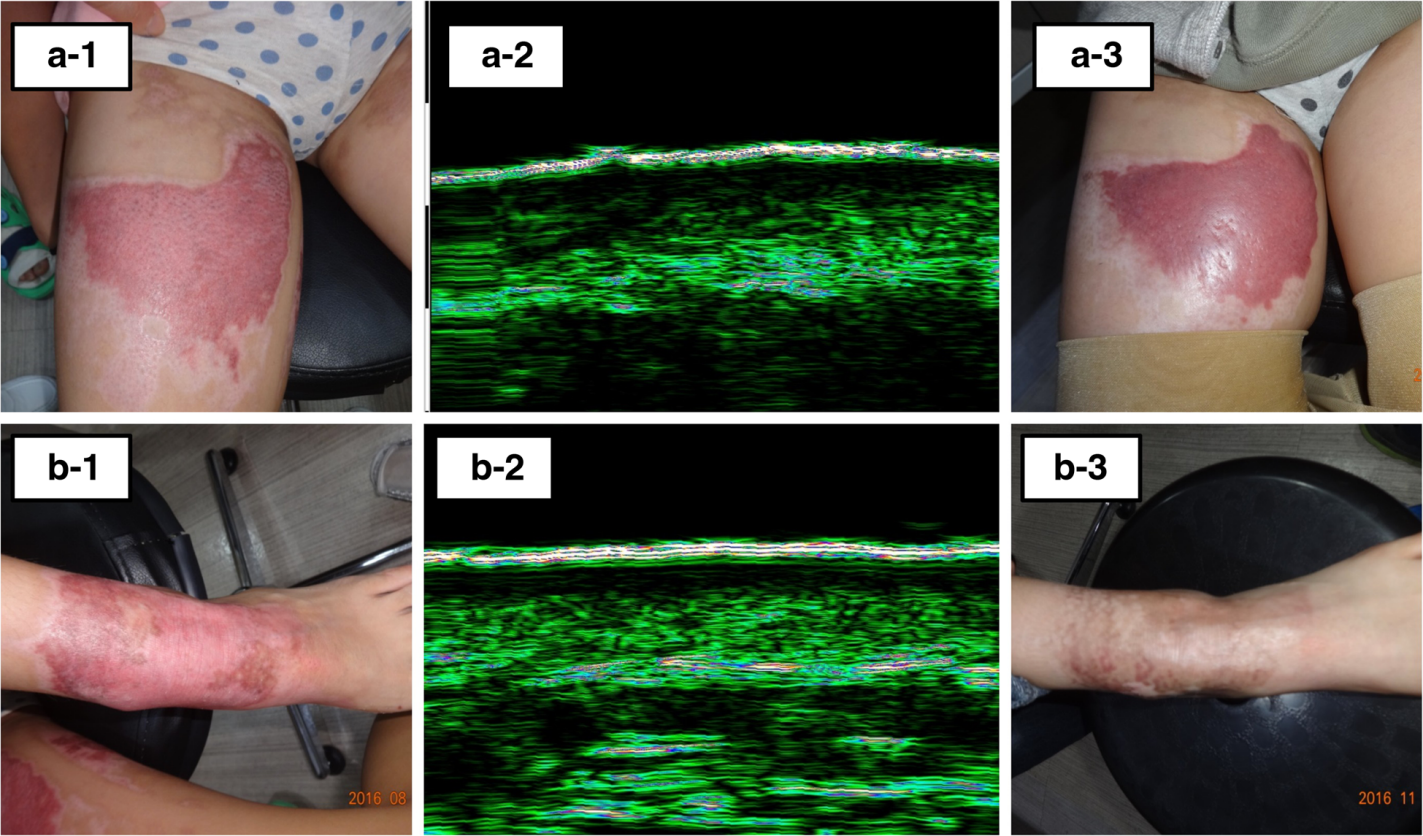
Clinical outcomes and initial ultrasound images of the scald burn in the same patient. The healing time of the right thigh was 24 days (a-1). The proportion of low-echogenic band (LEB) thickness was 35% (a-2), and a hypertrophic scar developed after 3 months (a-3). The healing time of the left ankle was 23 days (b-1). The proportion of LEB thickness was 25% (b-2), and normotrophic scar status was maintained (b-3)
